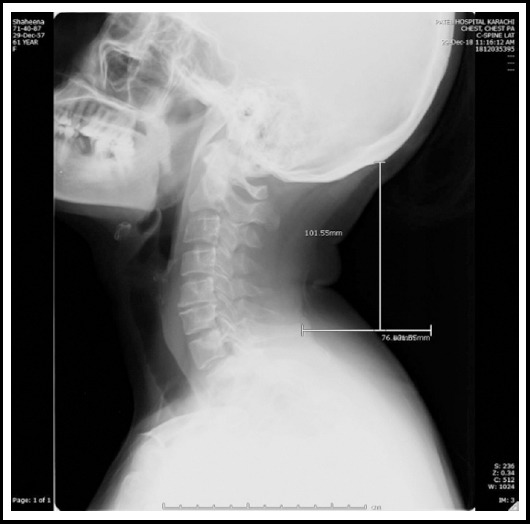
X-ray cervical spine (lateral view) demonstrating radiological parameters for measuring neck length on Synapse.
